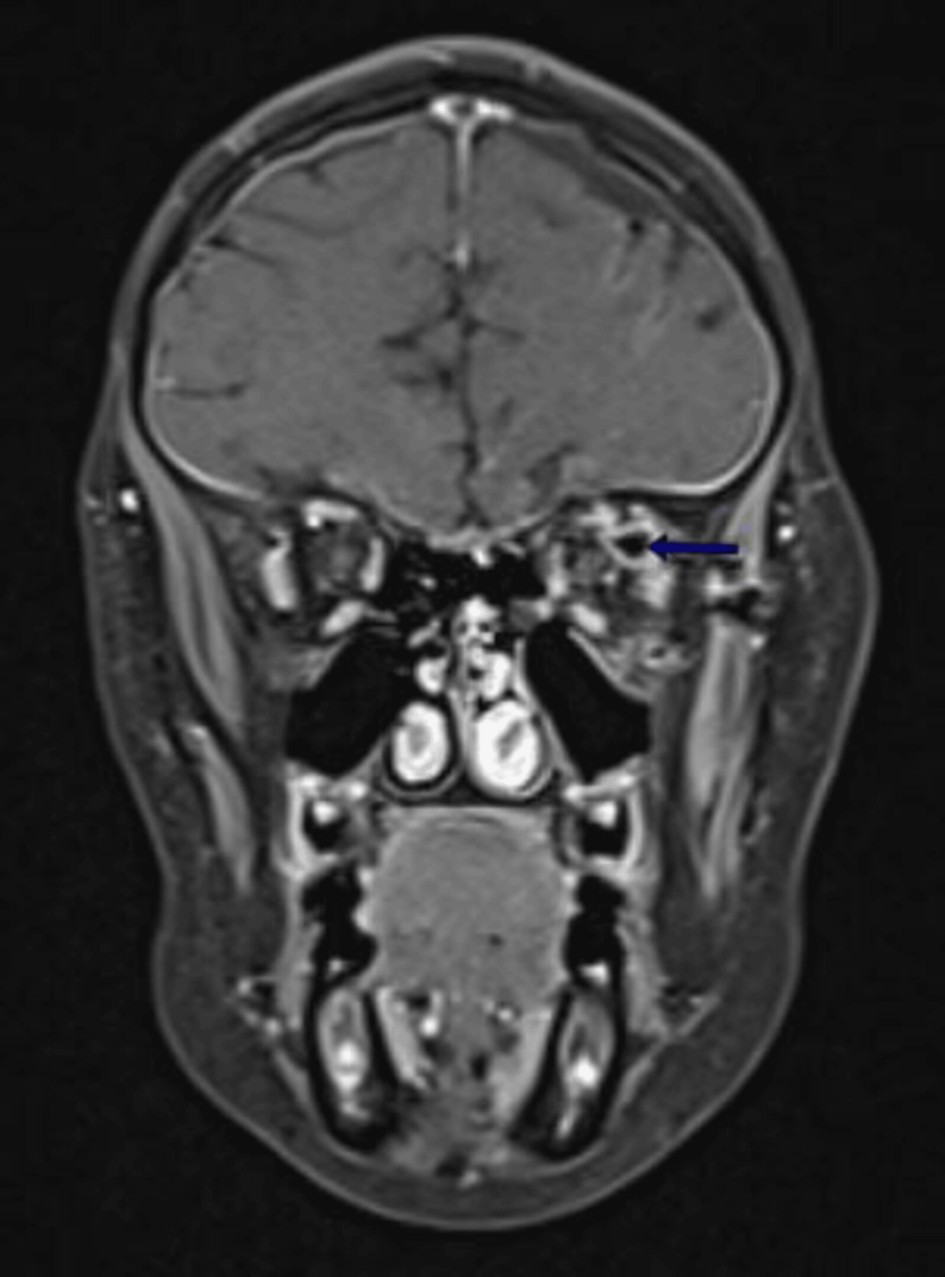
Coronal T1 fat-saturated (FAT SAT) MRI section after gadolinium injection, showing no enhancement of the mass (blue arrow). MRI, magnetic resonance imaging
